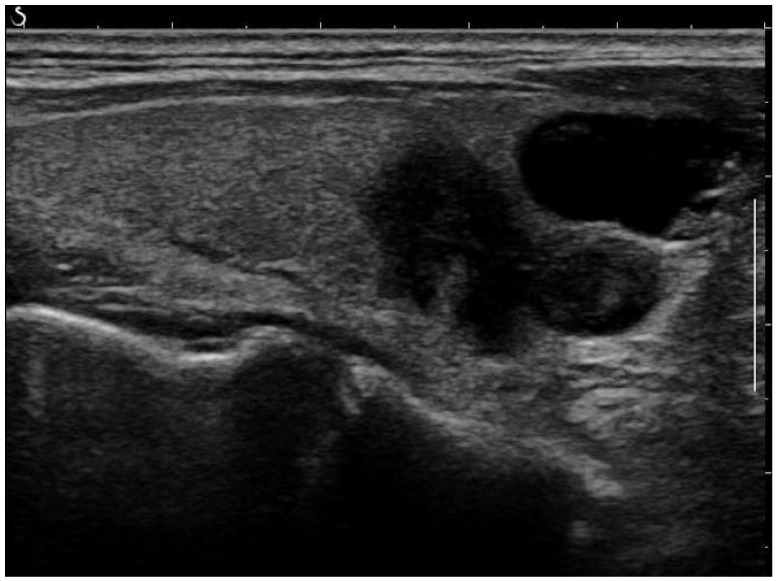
Raw ultrasound image of the thyroid nodule, with noise and artifacts covering part of the textures.
